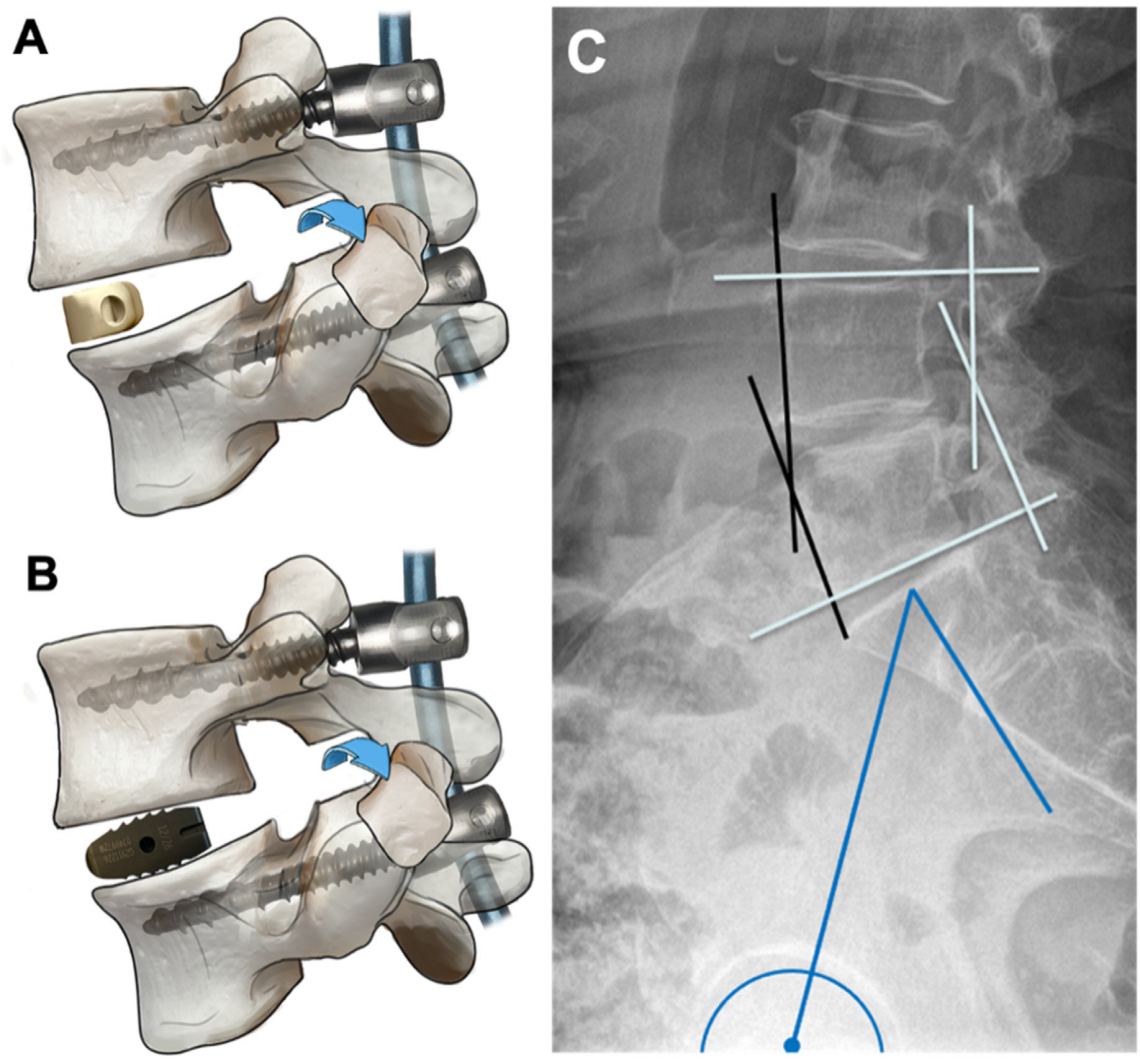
TLIF procedure and radiographic measurement. A, B. Dorsal release with bilateral facetectomy plus TLIF with insertion and rotation of a banana cage at the anterior vertebral edge (A) and plus TLIF with oblique insertion of a straight cage (B). C. Radiographic measurements. Cobb angle (white) was measured between lines paralleling the superior endplate of the upper vertebra and the inferior endplate of the lower vertebra. Anterior vertebral angle (AVB angle) (black) was measured between intersecting lines of the anterior surfaces of the vertebrae. The pelvic incidence (PI) (blue) is the angle between the perpendicular to the sacral plate at its midpoint and the line connecting the sacral plate midpoint to the center axis of the femoral heads. (For interpretation of the references to colour in this figure legend, the reader is referred to the Web version of this article.)
